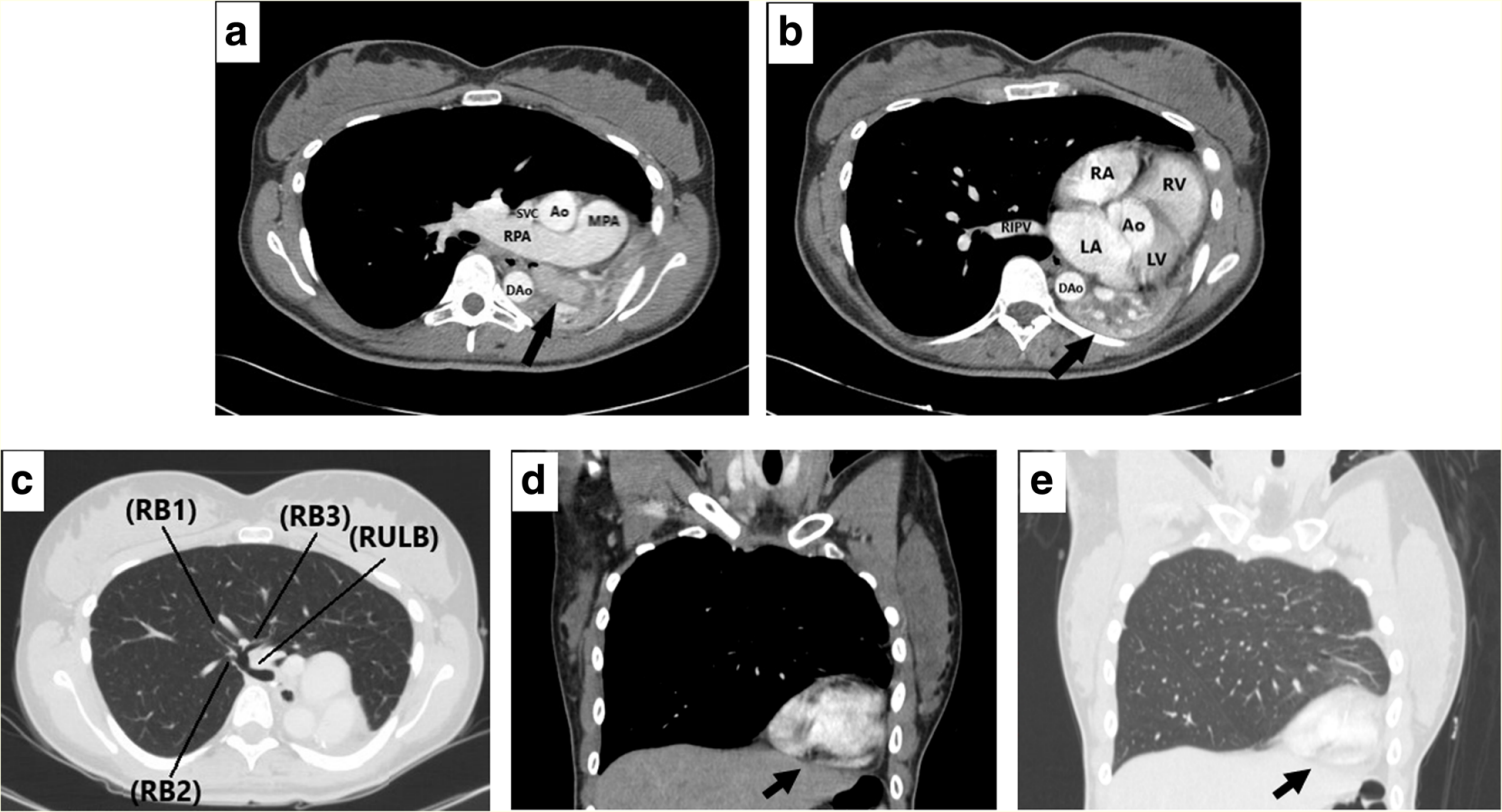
a. Axial contrast-enhanced chest CT scan with mediastinal-windowing shows an enhanced endoluminal mass within the left main bronchus (black arrow) and clockwise mediastinal shift. MPA, main pulmonary artery; RPA, right pulmonary artery; Ao, ascending aorta; DAo, descending aorta; RIPV, right inferior pulmonary vein. b. Axial chest CT scan with mediastinal-windowing demonstrates the completely collapsed left lung lying posterior to the heart (black arrow). Ao, ascending aorta; RA, right atrium; RV, right ventricle; LA, left atrium; LV, left ventricle; DAo, descending aorta. c. Axial chest CT scan section at the level of the right upper lobe imaged with lung window shows clockwise mediastinal shift with herniation of the right upper lobe into the left hemithorax. RULB, right upper lobe bronchus; (RB1), right upper lobe apical segmental bronchus; (RB2), right upper lobe posterior segmental bronchus; (RB3), right upper lobe anterior segmental bronchus. d. Coronal contrast-enhanced chest CT scan with mediastinal-windowing, shows extreme mediastinal shift towards the left and significant clockwise rotation of the heart (black arrow). e. Coronal contrast-enhanced chest CT scan imaged with lung window, shows overexpansion and substantial herniation of the right lung into the left hemithorax as well as severe clockwise rotation of the heart (black arrow)
